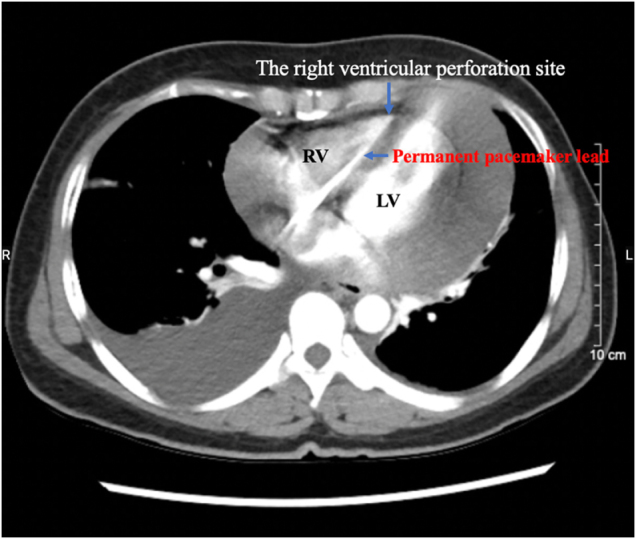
Contrast-enhanced computed tomography confirming the presence of pericardial effusion causing cardiac tamponade. The pacemaker lead is visualized perforating through the right ventricular wall.
